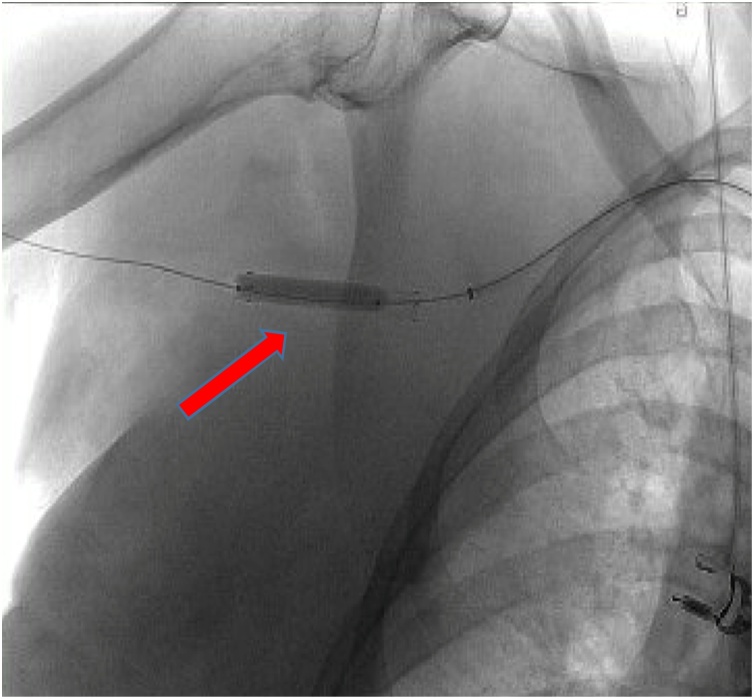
Intraoperative radiograph post-endovascular deployment of balloon-inflatable prosthesis over guide wire in distal axillary artery.
